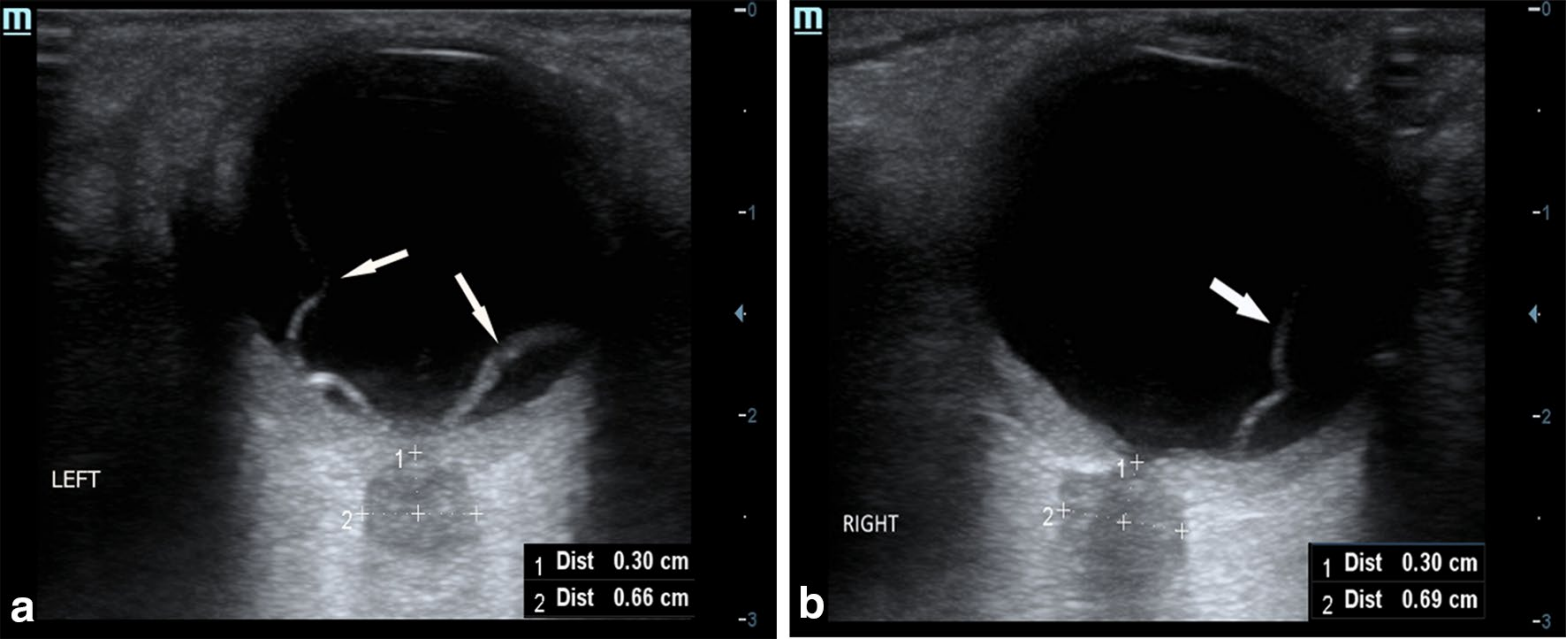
a Ocular ultrasound of the left eye demonstrating retinal detachment (white arrows) and optic nerve sheath diameter enlargement. b Ocular ultrasound of the right eye demonstrating retinal detachment (white arrow) and optic nerve sheath diameter enlargement
